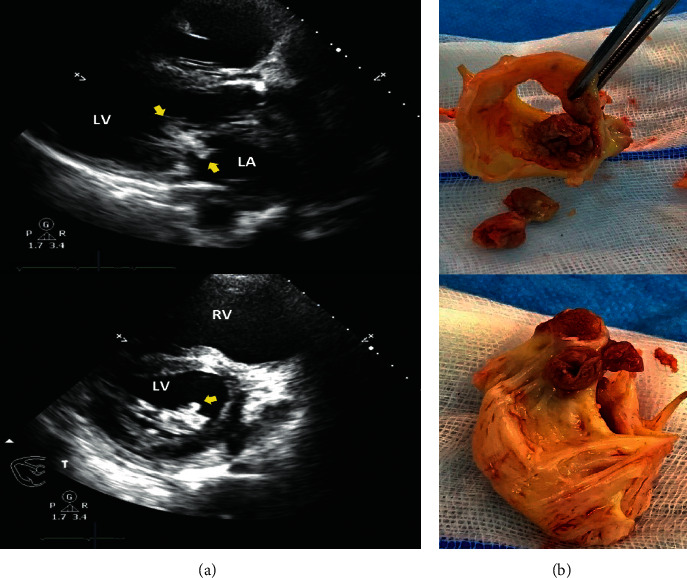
(a) Transthoracic echocardiogram showing multiple oscillating masses (yellow arrows) at anterior mitral valve leaflet with severe rheumatic mitral stenosis. (b) Gross findings of the resected mitral valve showing three large vegetations at anterior mitral valve leaflet. LV, left ventricle; LA, left atrium; RV, right ventricle.
